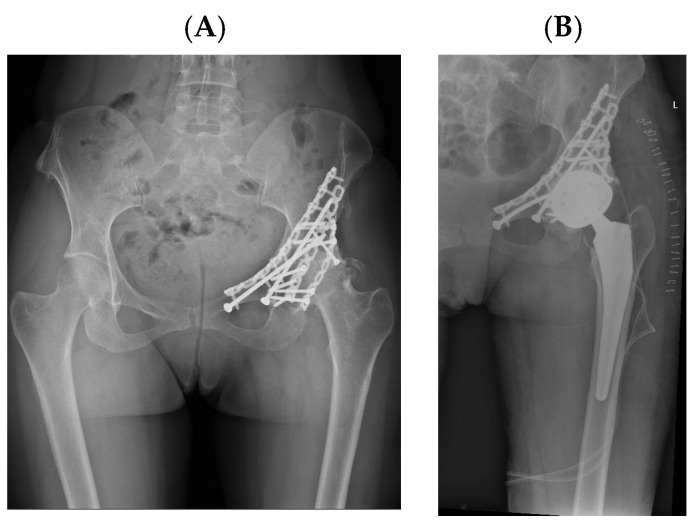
Post-traumatic osteoarthritis in an adult female patient following previous acetabular fracture fixation. (A) Preoperative radiograph; (B) postoperative radiograph after THA with a DM component.
